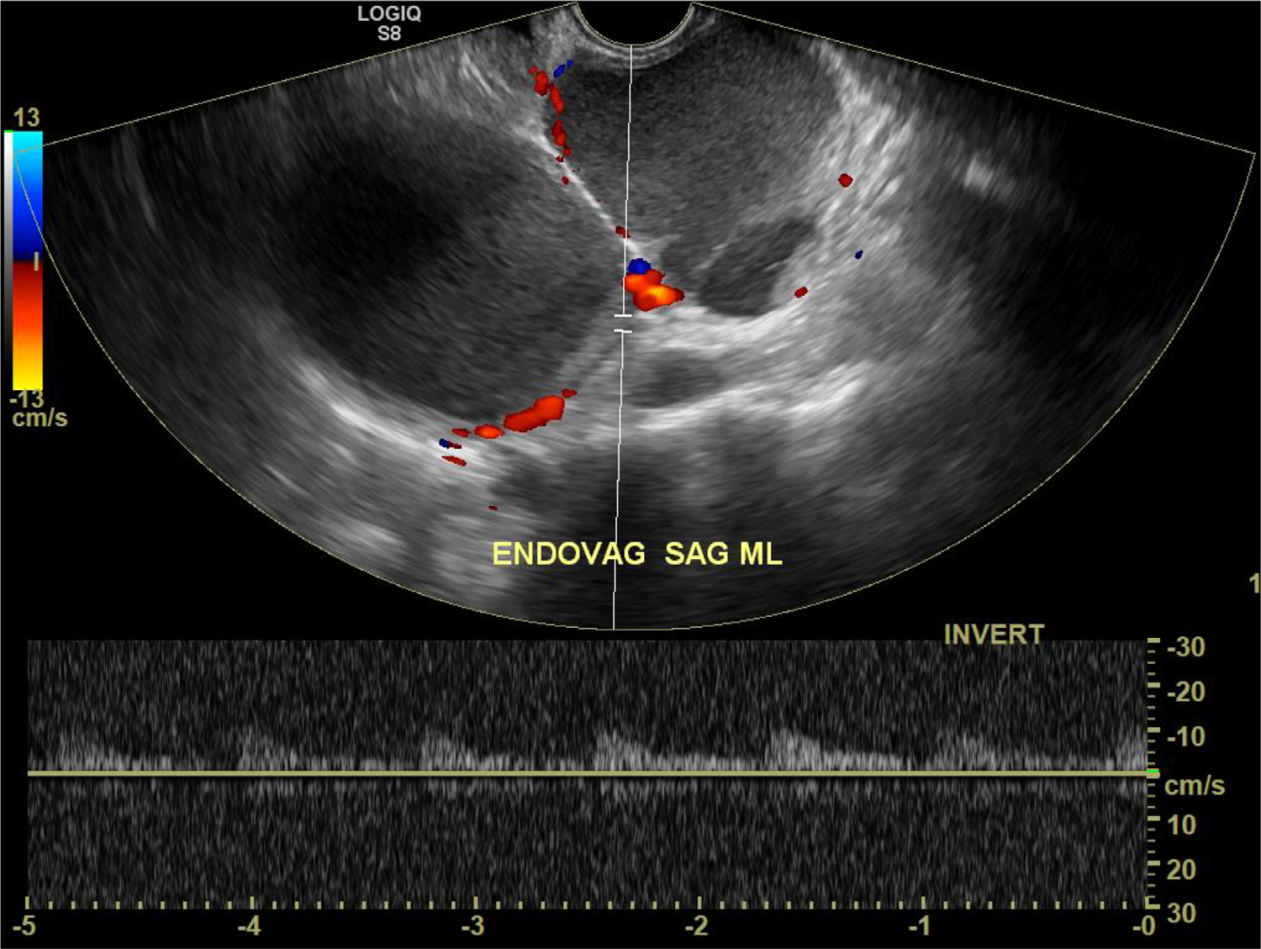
Transvaginal Ultrasound. Three well circumscribed, round, hypoechoic lesions are identified. The lesions contain complex fluid. Eco-color Doppler detected arterial-like blood flow within the walls of the lesion.
